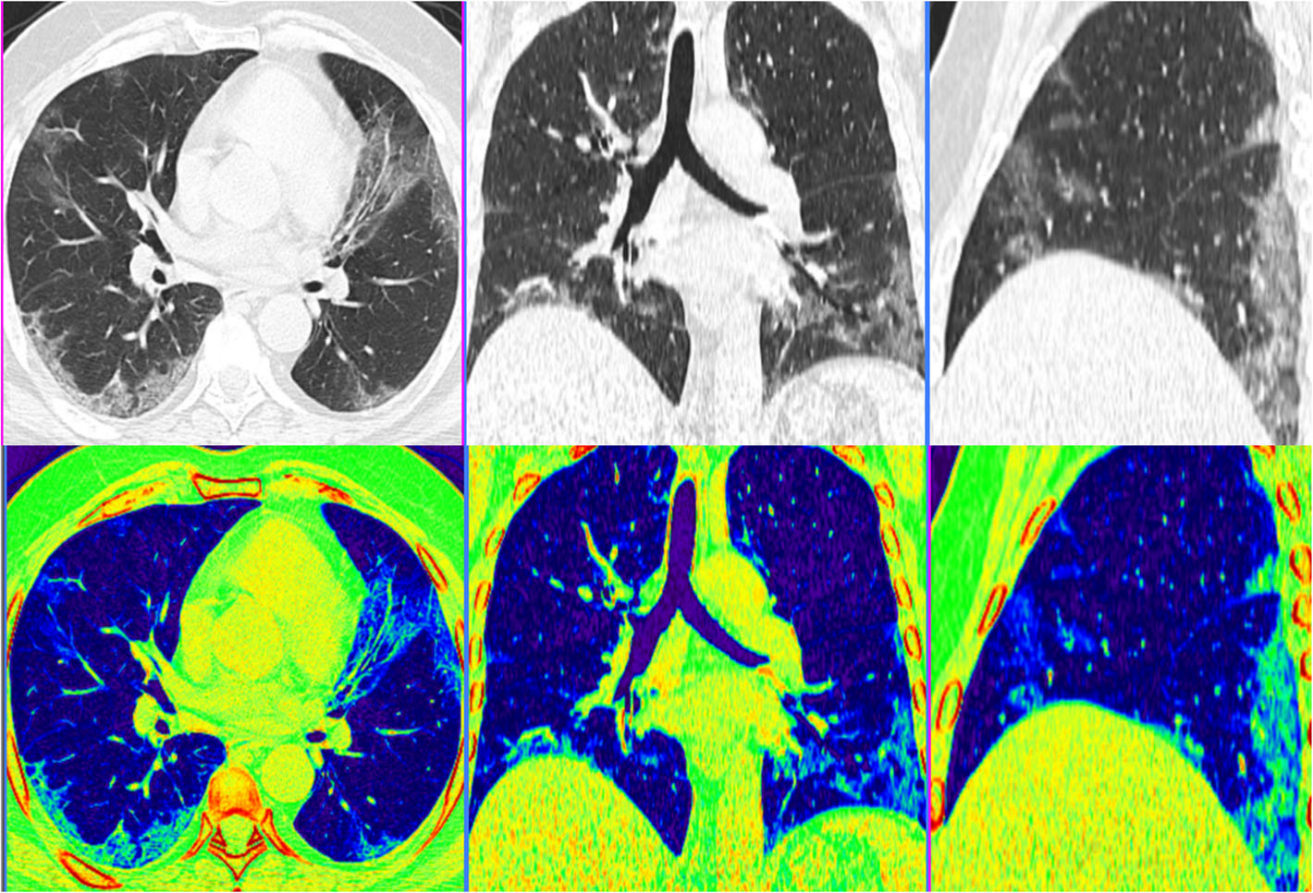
A 48-year-old male patient presented with fever and cough. MSCT scan with multi-planar and color-coded images showed multi-focal bilateral predominantly peripheral subpleural patchy ground glass opacities with septal and vascular thickening. CT-SSS was 11 and serum LDH was 239. The patient exhibited non-severe disease course
